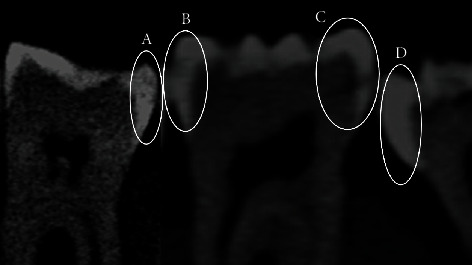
Sagittal CBCT images of the surfaces with different lesion depth and sound surface. (a) D1 Caries depth. (b) D2 Caries depth. (c) D3 Caries depth. (d) Sound surface.
